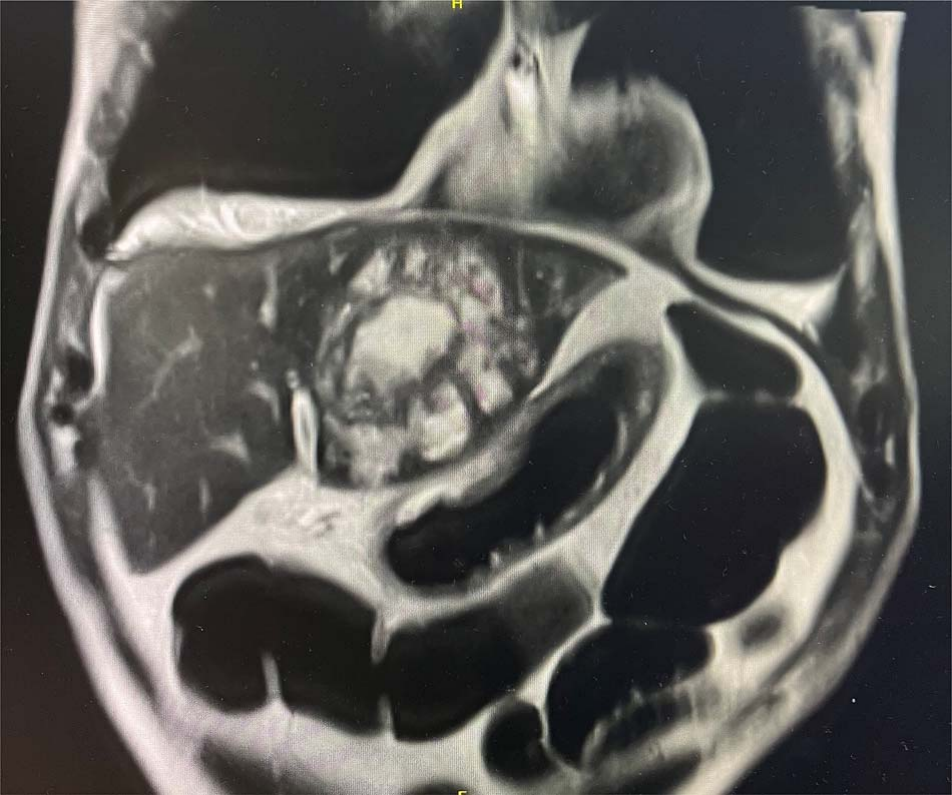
MRI liver in coronal slice. Hepatic abscess seen in left hepatic lobe.
